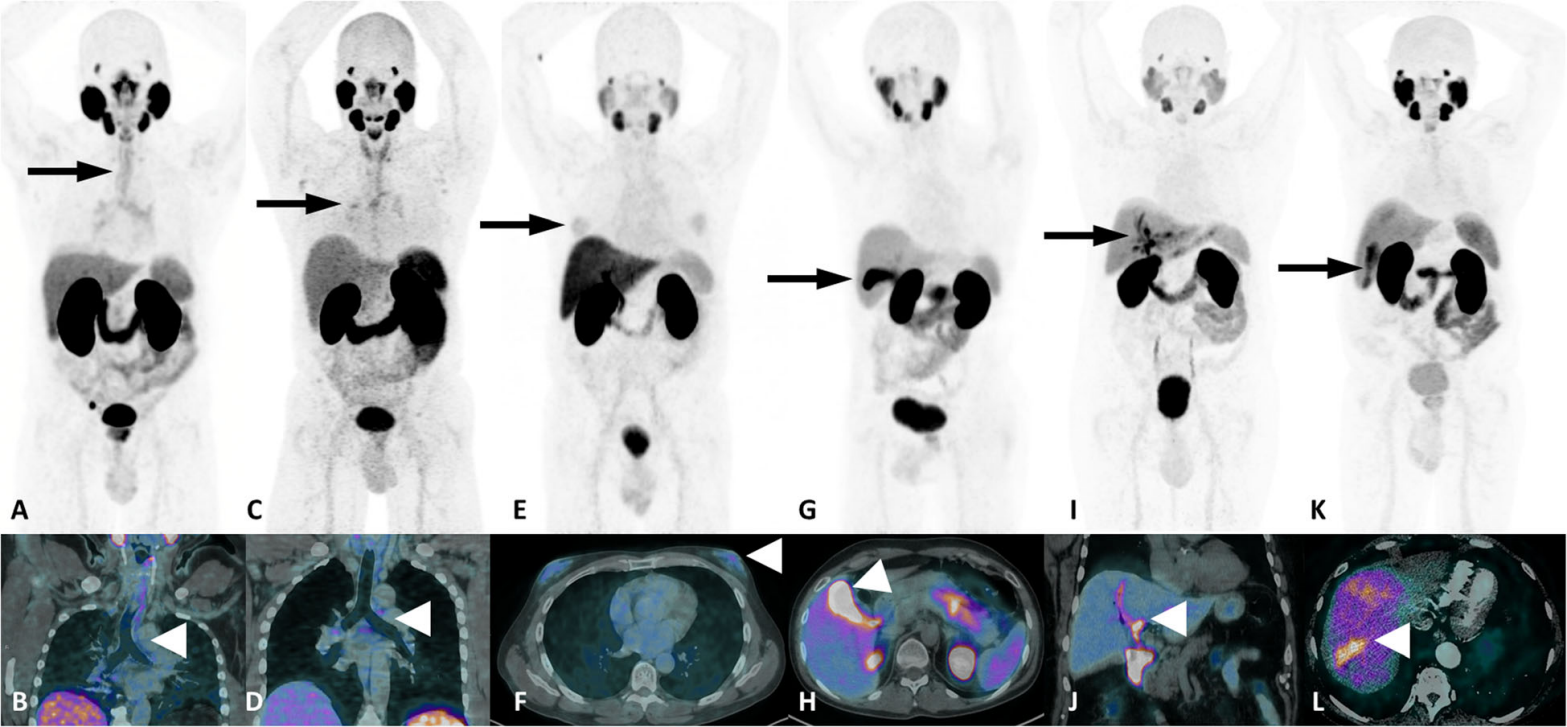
A miscelanea of normal variants of PSMA uptake. ^68^Ga-PSMA-PET MIP images on top (a, c, e, g, i and k) and corresponding fused PET/CT images on bottom (b, d, f, h and l) showing trachea and bronchi (a, b), mediastinal lymph nodes (c, d), gynecomastia (e, f), gallbladder (g, h), billiary tract (i, j) and liver perfusion defect (k, l)
